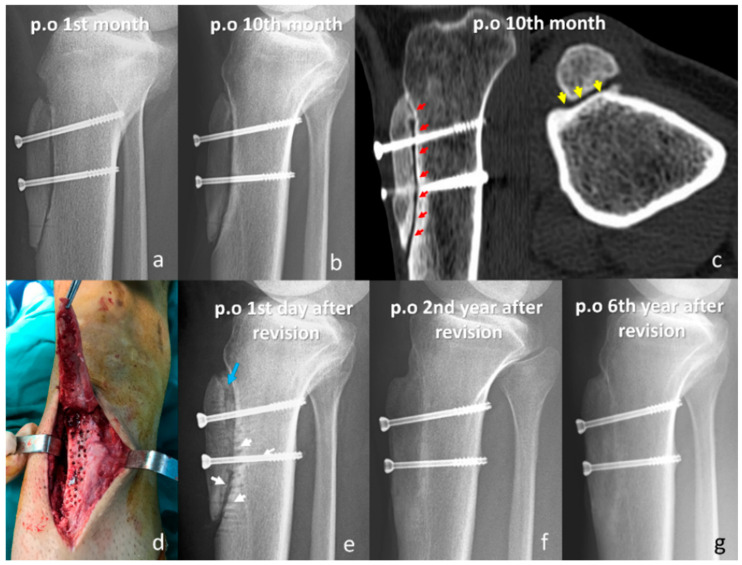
Nonunion case (a) Postoperative first-month lateral radiograph showing initial fixation with two-headed screws. (b) Tenth-month lateral radiograph indicating a persistent osteotomy line suggestive of nonunion. (c) Coronal and axial CT images at 10 months postoperatively demonstrating sclerotic bone edges and radiolucent lines (red arrows) at the osteotomy site, consistent with nonunion. The axial view also shows the established nonunion with a sclerotic osteotomy plane (yellow arrows). (d) Intraoperative photograph taken during revision surgery showing fibrous tissue at the osteotomy site and sclerotic bone requiring debridement. (e) Immediate postoperative radiograph following revision with autologous iliac crest bone grafting and screw refixation; the blue arrow indicates the autograft, and white arrows show the multiple drilling sites. (f) Follow-up radiograph at two years post-revision showing complete union at the osteotomy site. (g) Six-year follow-up radiograph demonstrating maintained union and stable fixation without further complications.
